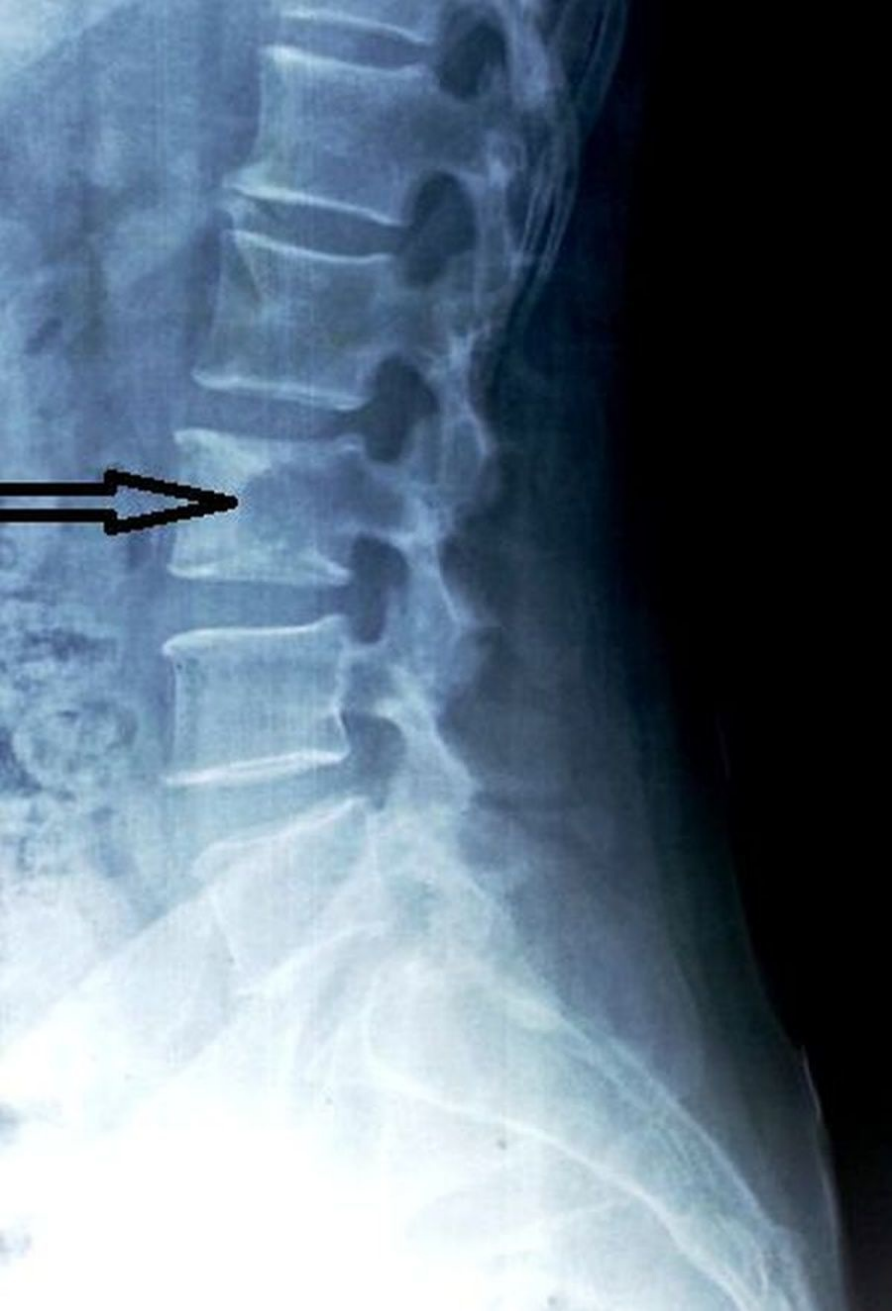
Plain radiograph, lateral view of the lumbosacral spine showing an osteolytic lesion in the L-3 vertebral body.
